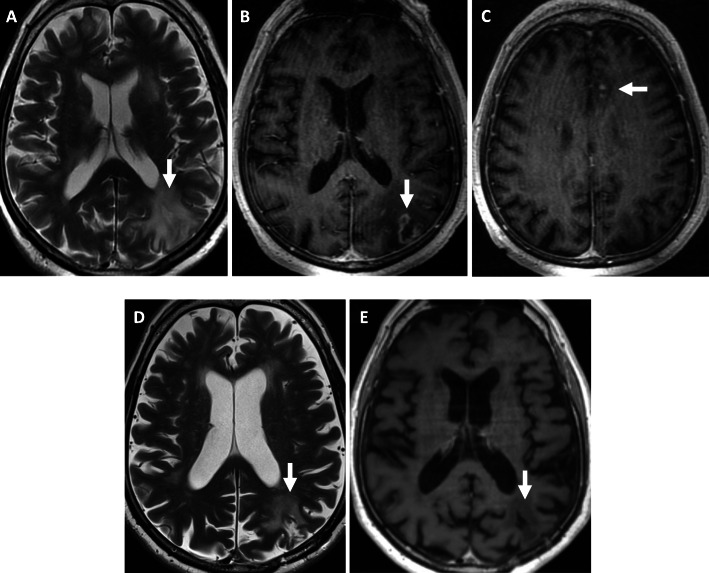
a Axial T2-weighted and b axial T1-weighted post-contrast images show a new rim-enhancing mass with vasogenic oedema in the left parietal lobe (arrow) and c patchy cortical enhancement in the left cingulate gyrus (arrow). Post-treatment MRI brain d axial T2-weighted and e T1-weighted post-contrast images show decrease in vasogenic oedema and reduced enhancement in the left parietal lobe compatible with treatment response
